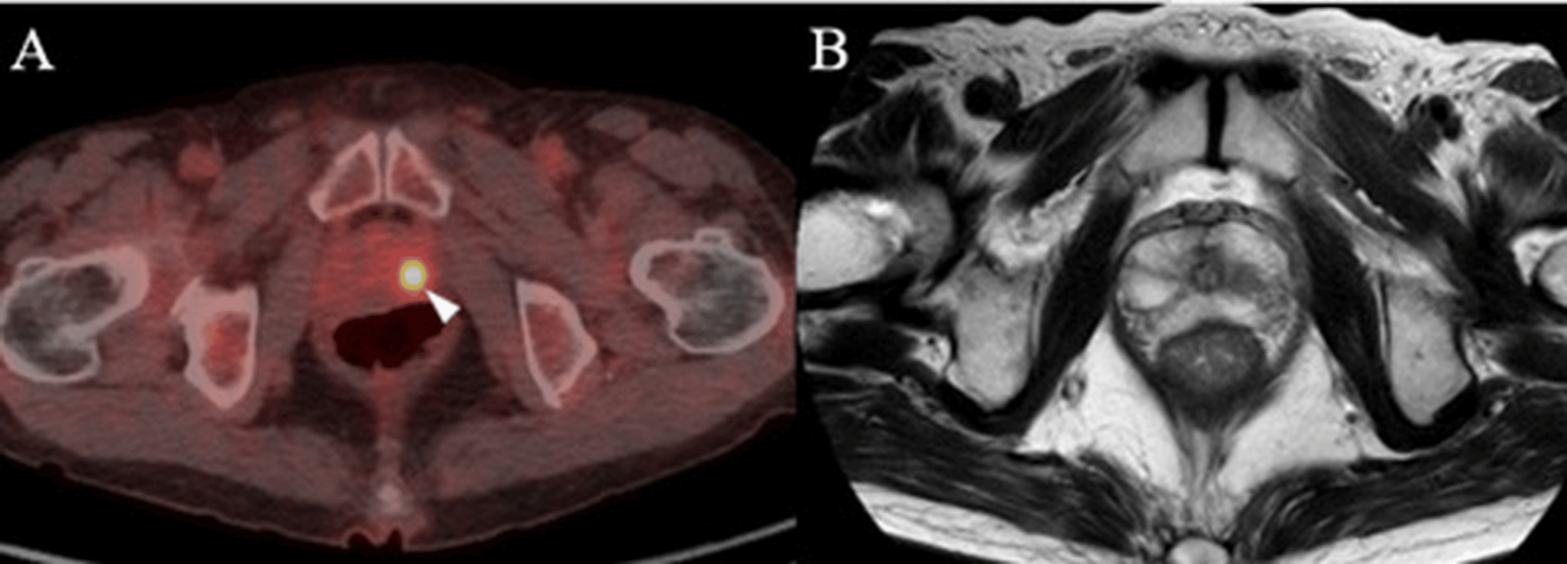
The images of prostate cancer 2-Deoxygenated-2-fluoro-D-glucose positron emission tomography-computed tomography of the whole body showed a hyperintense lesion in the left lobe of the prostate (A), while magnetic resonance imaging showed a hypointense lesion in the same area (B). Prostate biopsy revealed a diagnosis of prostate cancer.
